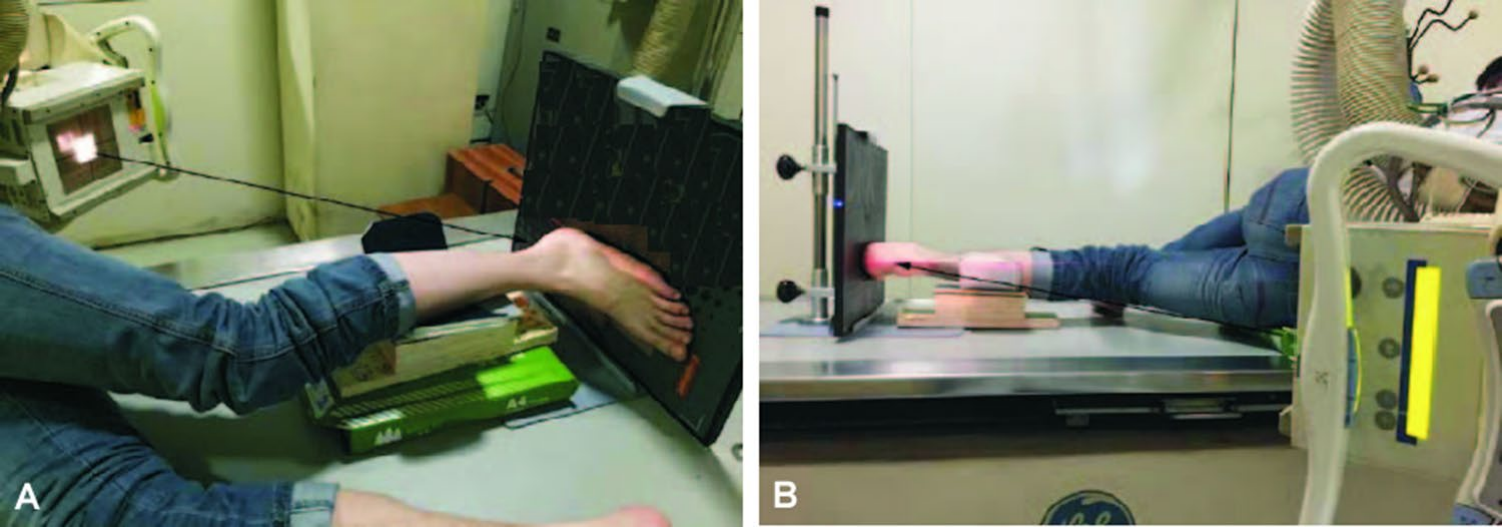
Modified horizontal calcaneal axial radiography from different perspectives.
